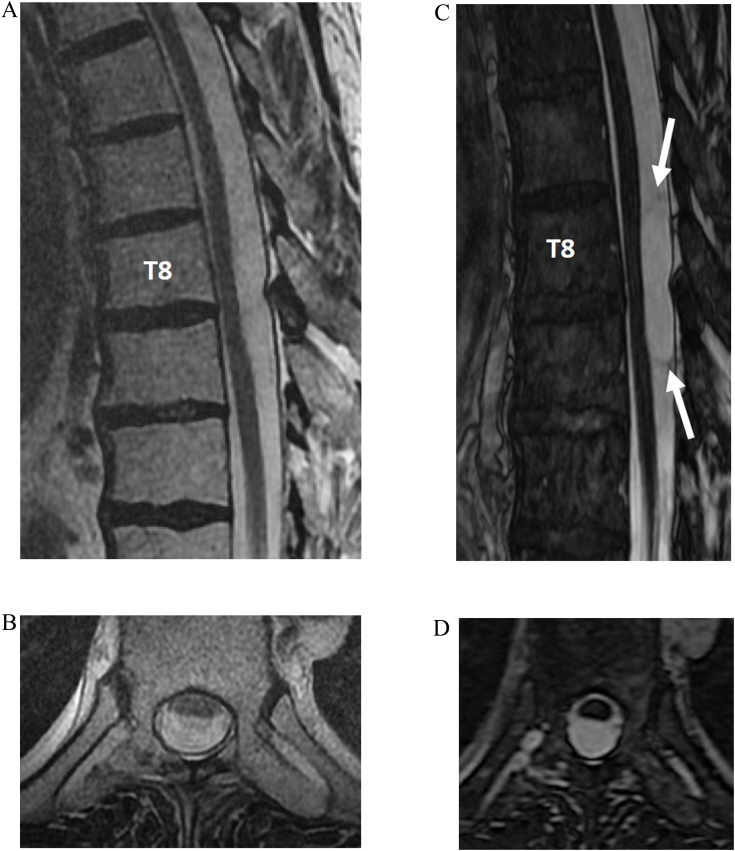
Intradural arachnoid cyst. Sagittal and axial T2-weighted MRI images (slice thickness, 3 mm) (a–b) demonstrate a subtle ventral displacement of the thoracic spinal cord. No definable lesions are visualized. Sagittal and axial FIESTA-C MRI images (c–d) demonstrate the delicate walls of the arachnoid cyst, as well as the homogenous signal intensity of the cyst contents.
